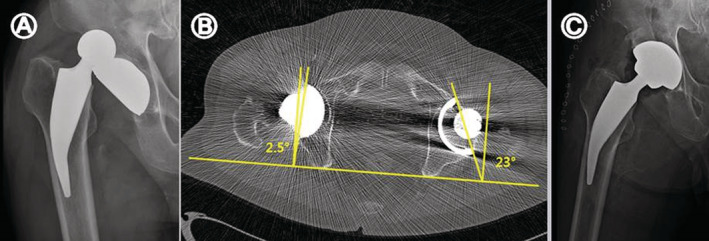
(A) Radiograph of a 66‐year‐old man with recurrent dislocation of implant at 2 weeks postoperatively. (B) Axial CT scan image demonstrating the decreased acetabular component anteversion of right hip (2.5°). (C) Revisional replacement of acetabular cup was done 7 months after the primary surgery.
